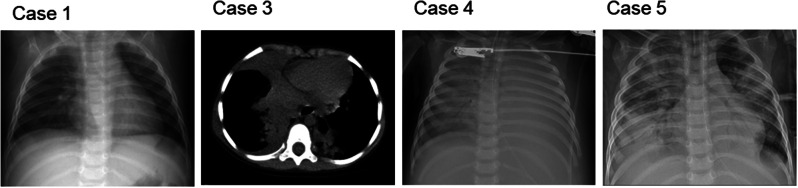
Pulmonary imaging of patients 1, 3, 4, and 5. Patient 2 underwent X-ray examinations at an external hospital and no imaging data were acquired
